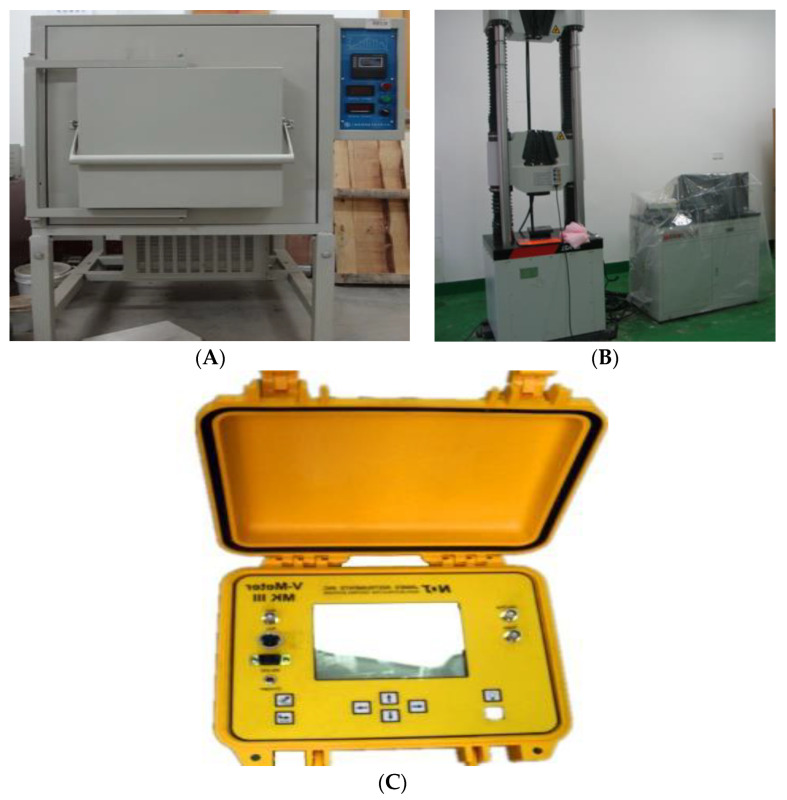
Test equipment. (A) SMF1900-50 Chamber Resistance Furnace. (B) Rigid Servo Triaxial Compression Tester. (C) 2V-METER III Ultrasonic Pulse Velocity Tester.
